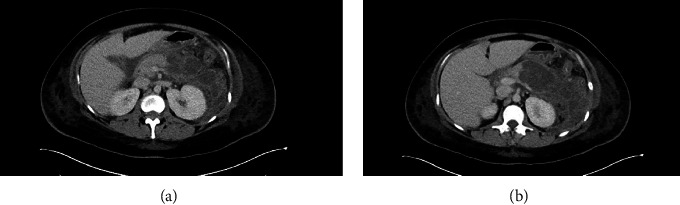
(a, b) CT scan showing the pancreatic fluid collection measuring 13.2 × 5.5 cm in cross-section and 12.8 cm in craniocaudal length.
